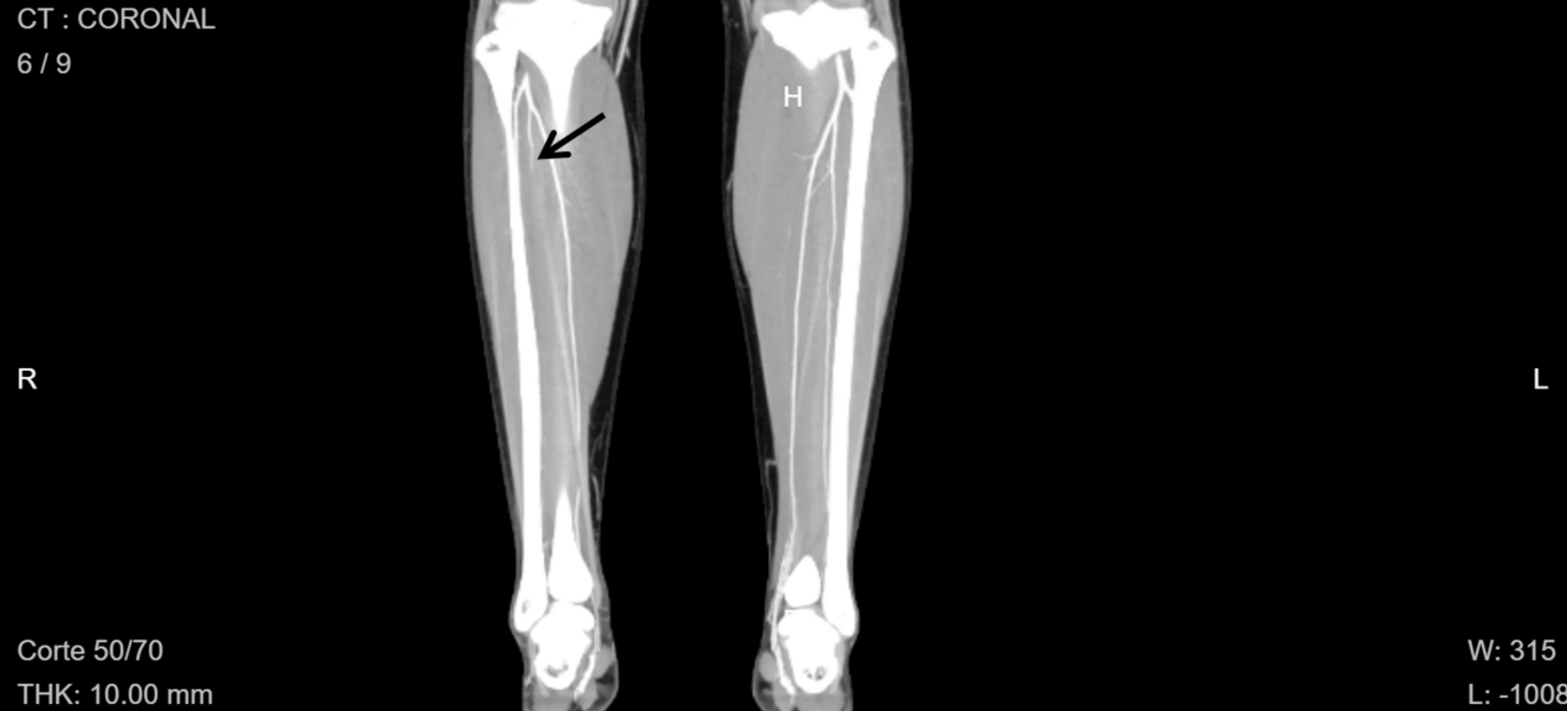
Coronal computed tomography image of the lower limbs. The arrow indicates occlusion of the proximal segment of the right peroneal artery.
